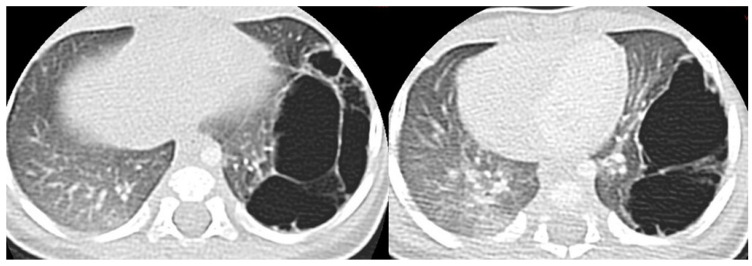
A CT scan performed one month later in the same patient as in Figure 3, showing multiple large air-filled cysts involving the left lower lobe, with a maximum diameter of 60 mm, confirming the diagnostic hypothesis of type 1 CPAM.
